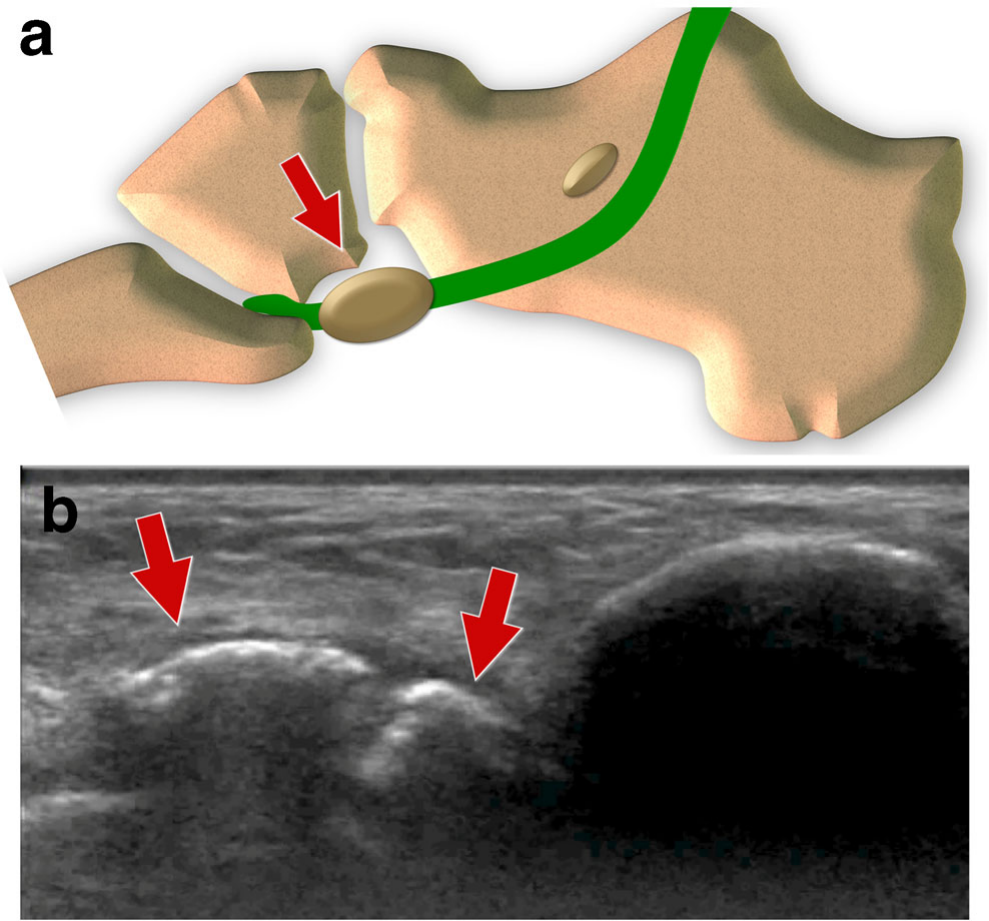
Hypertrophic os peroneum. *7A* Scheme *7B* Ultrasound. Hypertrophic OP facilitated a local impingement
